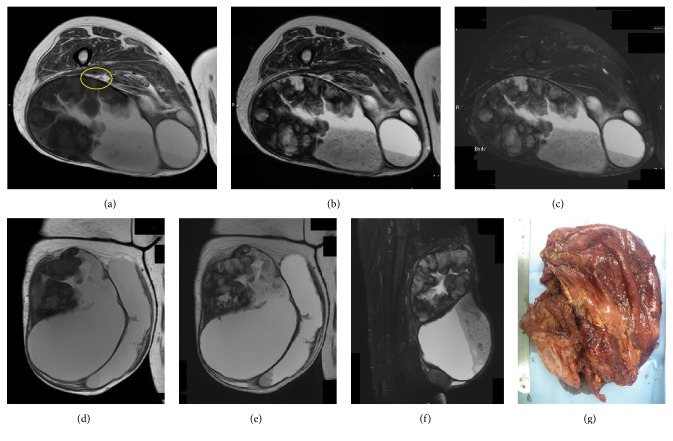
69-year-old female with a chronic expanding hematoma in the deep thigh. MRI shows a huge lesion with cystic features ((a–c) axial image; (d-e) coronal image; (f) sagittal image). The sciatic nerve where it branches into the tibial nerve and the common peroneal nerve is not obvious on axial section. A yellow circle indicates the area where the nerve is supposed to exist (a). MRI shows heterogeneous low to slightly intermediate intensity on T1-weighted imaging (a, d). Heterogeneous low to high signal intensity is seen on T2-weighted (b, e) and fat-suppression T2-weighted imaging (c, f). A fibrous cystic wall is visible after removal of the hemorrhagic coagulation material (g).
